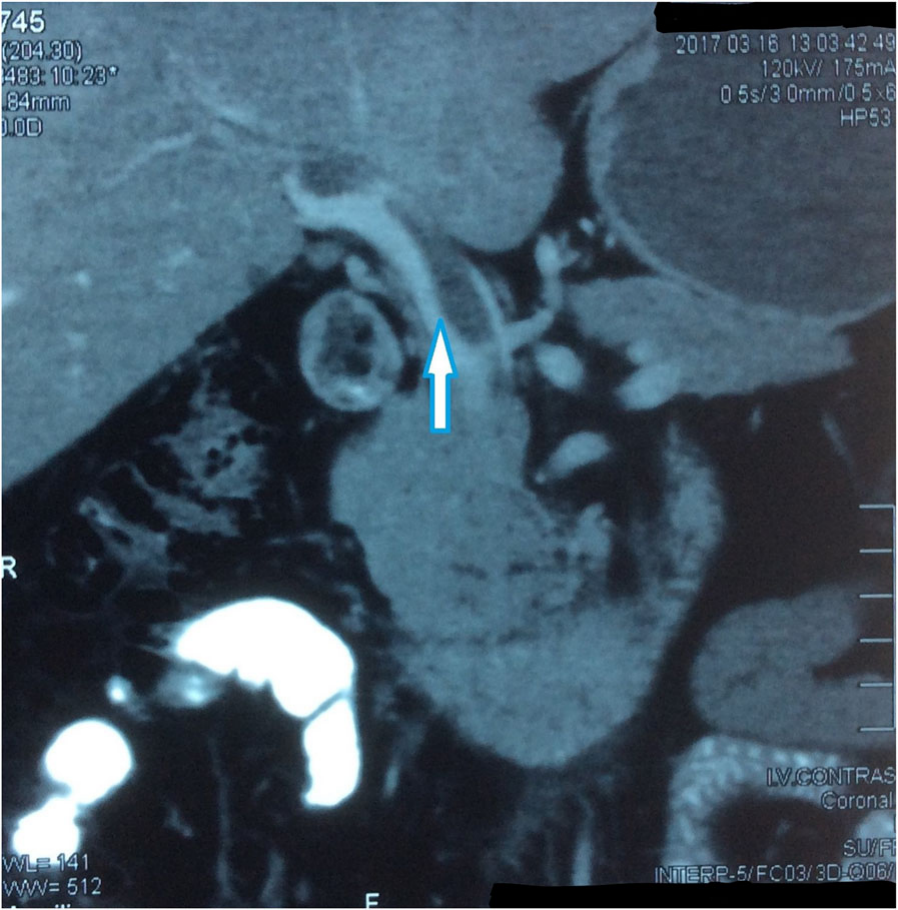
Contrast-enhanced computed tomography abdomen coronal sections. Thrombus in the portal vein (*white arrow*)
